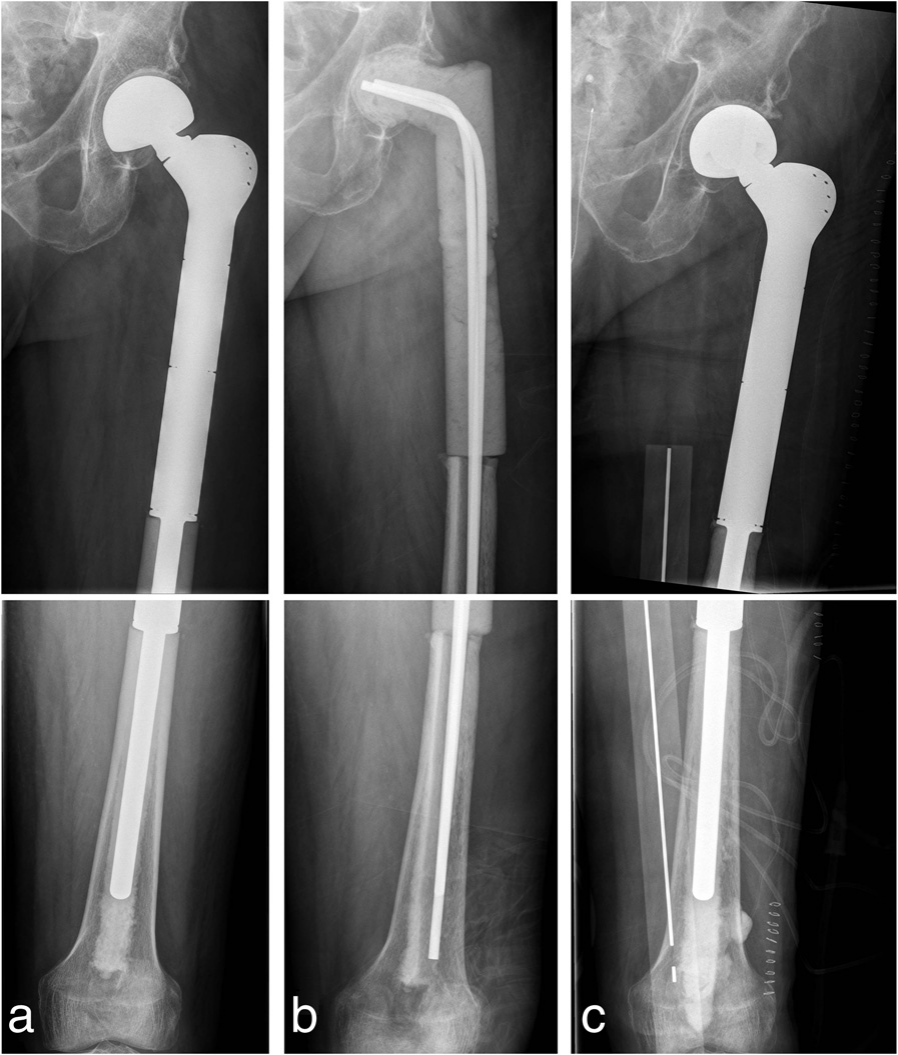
a Anterior-posterior X-ray of infected femur replacement (chronic infection); b After removal and with inserted cement spacer; c After reimplantation of a proximal femur replacement
